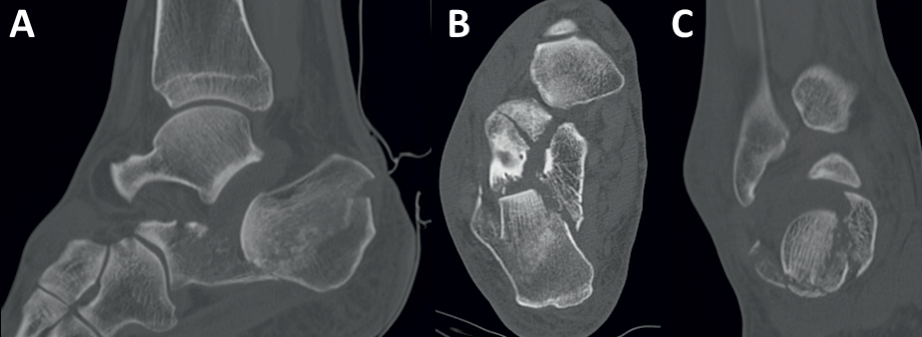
CT scan images of the sagittal (A), axial (B), and coronal view (C) of the fracture at the point of depression of the posterior facet.
